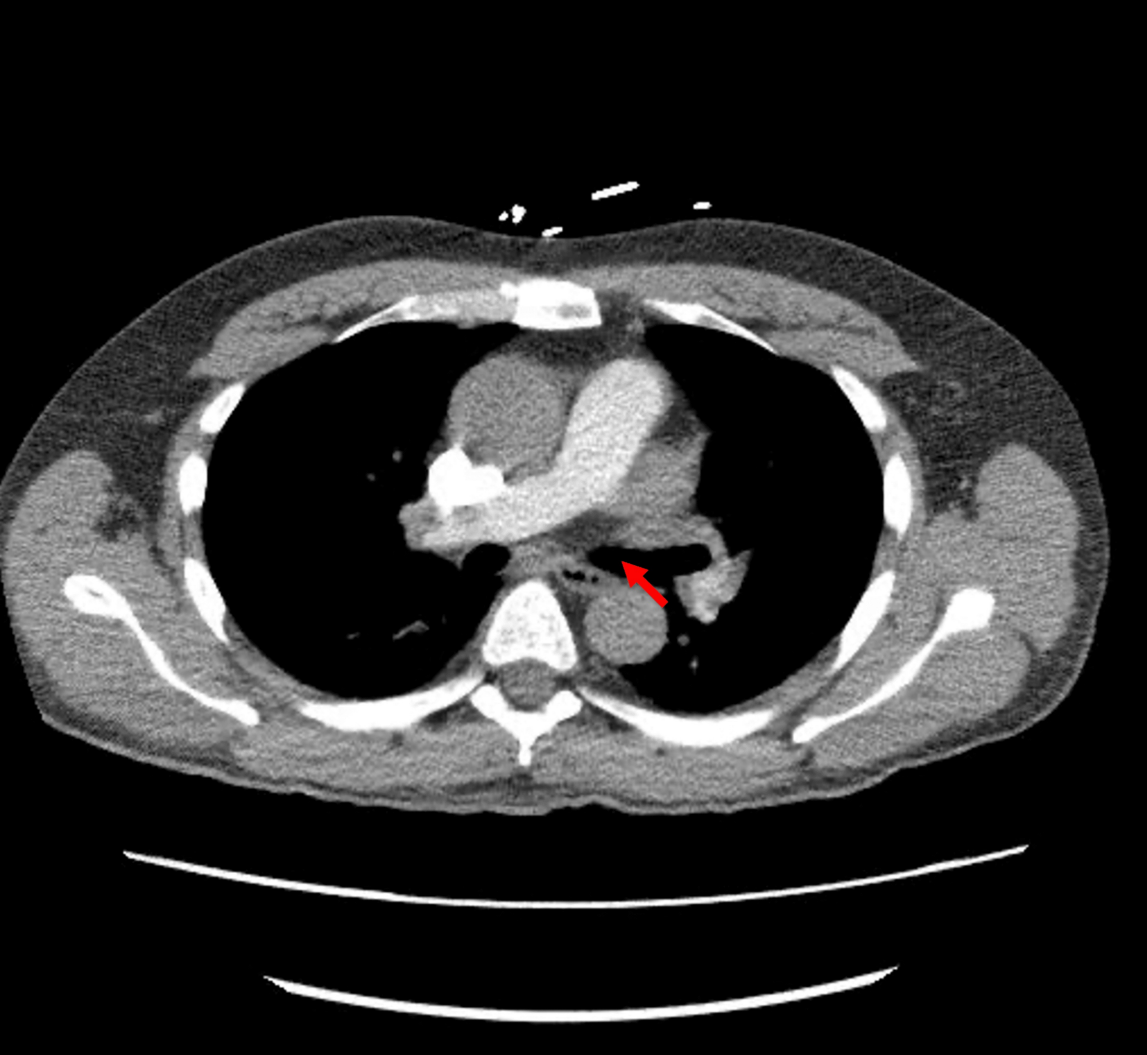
CT pulmonary angiogram demonstrating left lower lobe pulmonary embolism. This is an axial slice of a contrast-enhanced CT pulmonary angiogram at the level of the lower pulmonary arteries. The red arrow indicates a filling defect in the left lower lobe pulmonary artery, consistent with an acute pulmonary embolism. Note the central lucency within the contrast-opacified vessel, indicative of the embolus.
